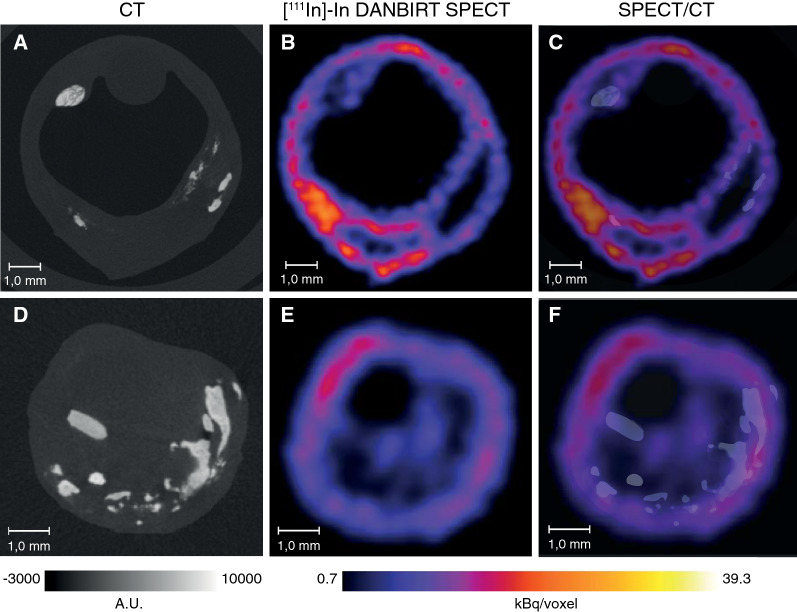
CT images (A, D) and corresponding SPECT images of uptake for [^111^In]In-DANBIRT (B, E) with overlay image (C, F) of two typical examples (1 A-C, 2 D-F). *A.U*, arbitrary units
